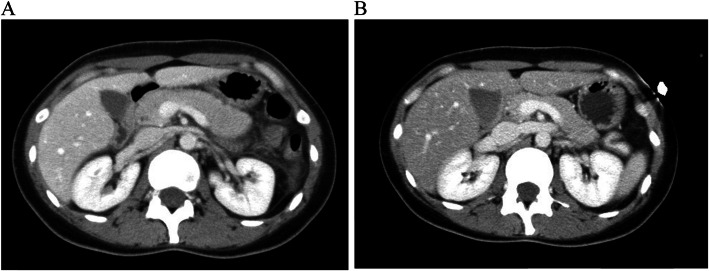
Abdominal CT scans. a: At the local hospital, imaging studies revealed swelling of the pancreas and mild ascites. b: On admission to our hospital, no abnormalities were detected. CT: computed tomography
